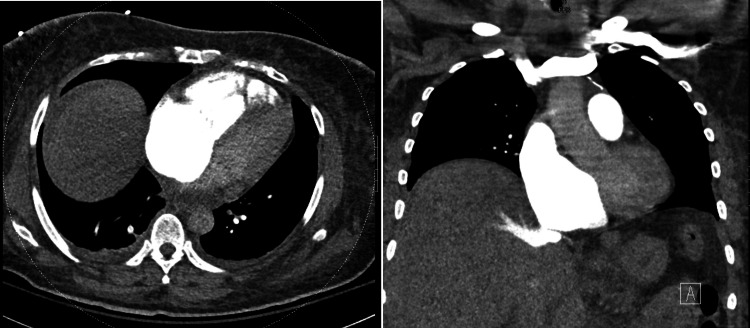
Preoperative CT showing enlarged RV CT: computed tomography, RV: right ventricle
